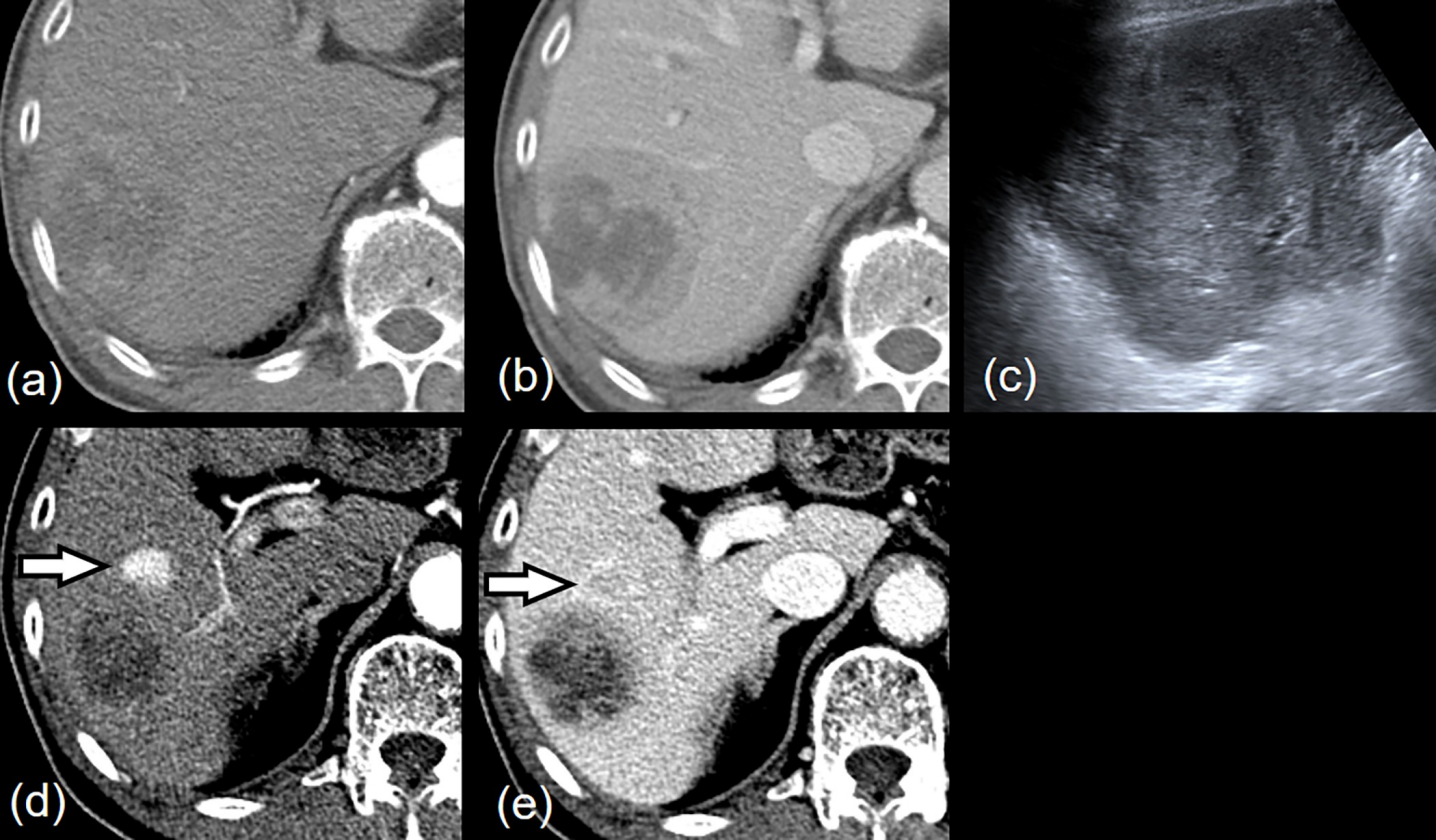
Discrepancy in a case between preliminary and final interpretations confirmed hepatocellular carcinoma. A 79-year-old man showed fever and right flank pain. (A, B) CT images show thick rim enhancing mass in the right posterior section of the liver. The heterogeneously enhancing mass in the liver was mistaken for liver abscess by the on-call resident. (C) The lesion shows a heterogeneous echoic mass in abdominal ultrasonography. (D) Another small arterial enhancing nodule in the inferior aspect of the lesion with washout (E) in the portal venous phase. Attending radiologist reported HCC in the right posterior section with additional HCC. A subsequent biopsy revealed HCC.
